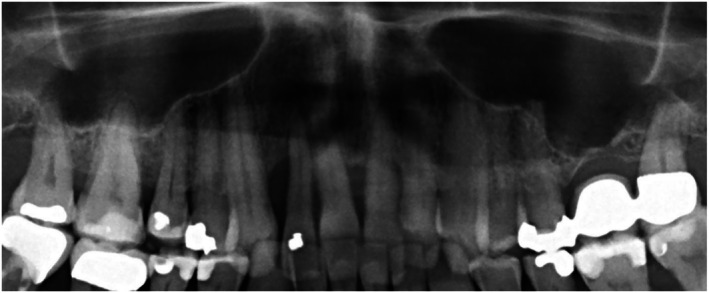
Initial panoramic radiograph showing the maxillary section of the orthopantomogram (OPG).
